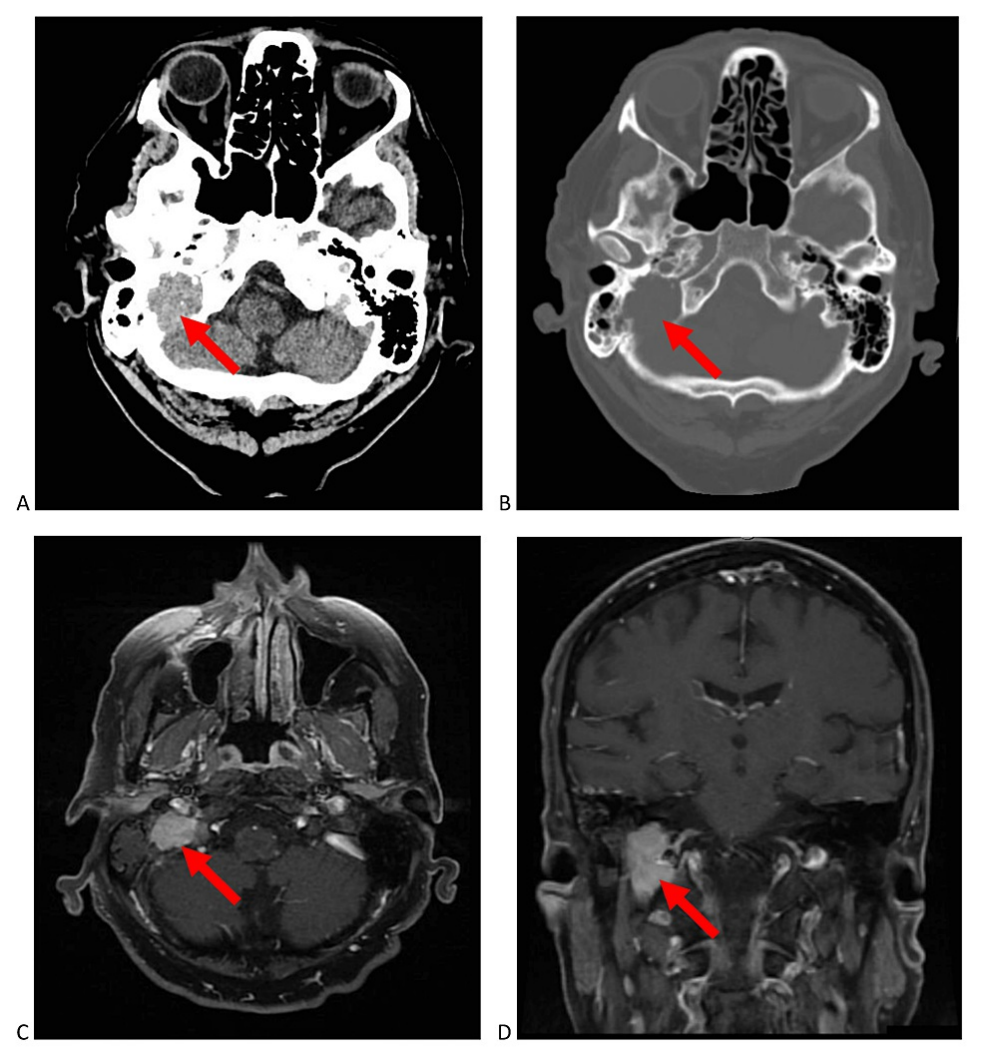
Brain imaging of the tumour arising in the right jugular foramen at presentation. (A) soft tissue axial non-contrast computed tomography (CT) and (B) bone-window axial non-contrast CT showing an expansile mass in the right jugular foramen causing osteolysis and “moth-eaten” borders of the adjacent petrous bone. (C) axial and (D) coronal T1-weighted post-contrast magnetic resonance imaging (MRI) showing marked uniform enhancement of the right jugular foramen mass. The superior extent of the tumour is just inferior to the vestibular apparatus.
